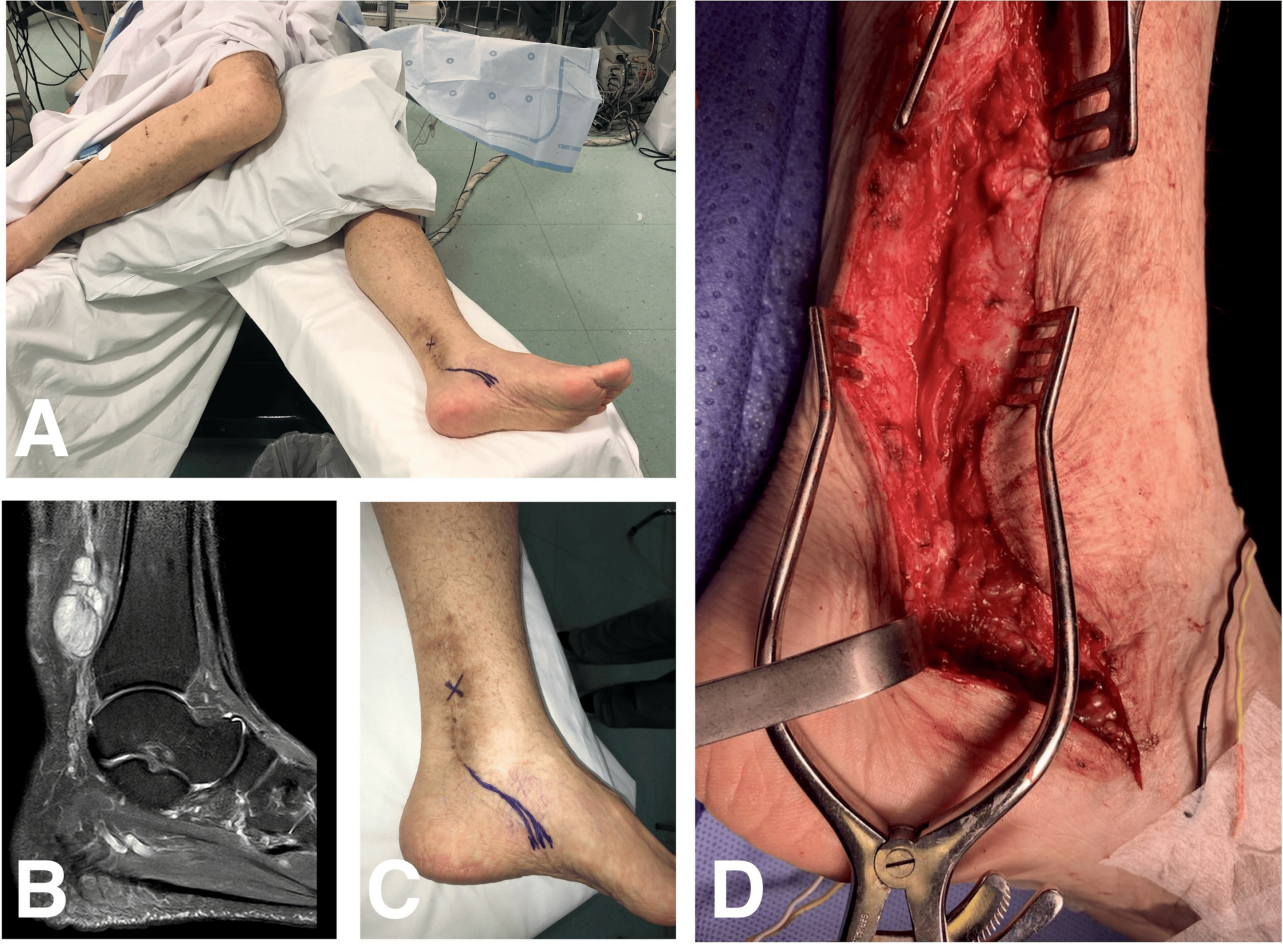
Case 3: Intraoperative photographs showing surgical positioning and planned skin incision, as well as the final aspect of tarsal tunnel decompression (A, C, and D); sagittal T2-weighted MRI of the left leg three years before the current admission demonstrating a distal leg tibial nerve schwannoma outside the tarsal tunnel (B).
